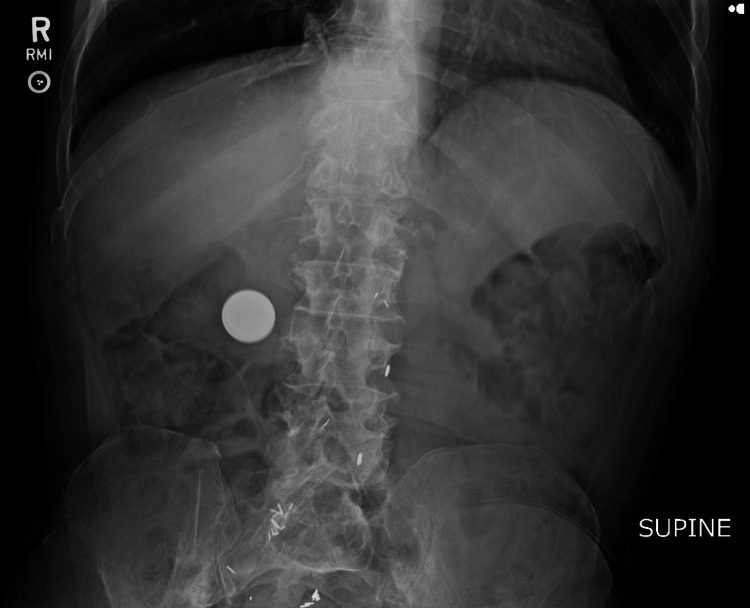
Abdominal X-ray Foreign body in the distribution of the distal stomach.
